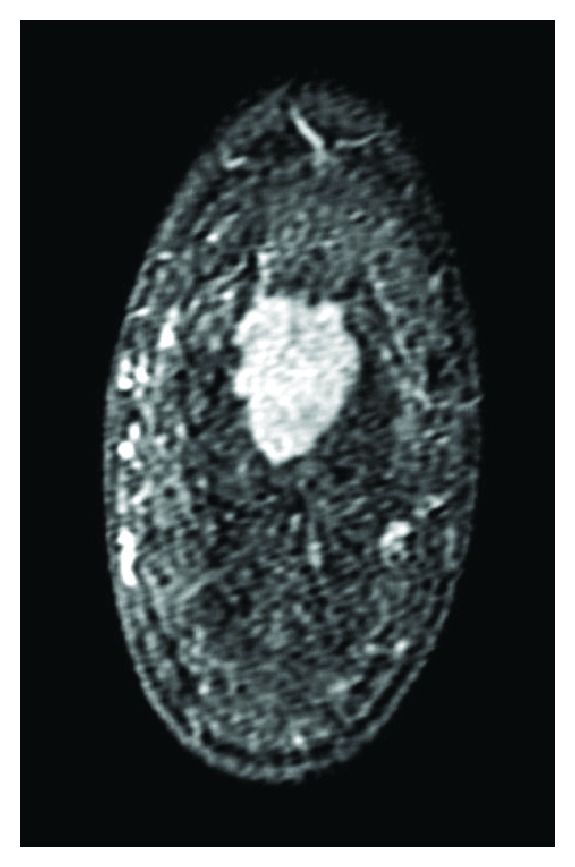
Enhanced MRI revealed a mass of high intensity, but no intraductal spread was detected (coronal section).
